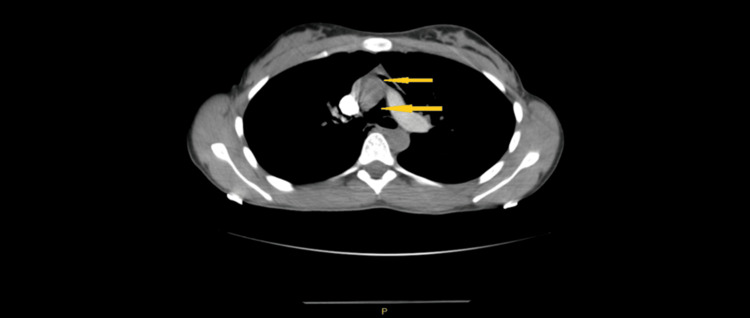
CT pulmonary angiogram showing the pneumomediastinum Yellow arrows indicate air in the mediastinum
